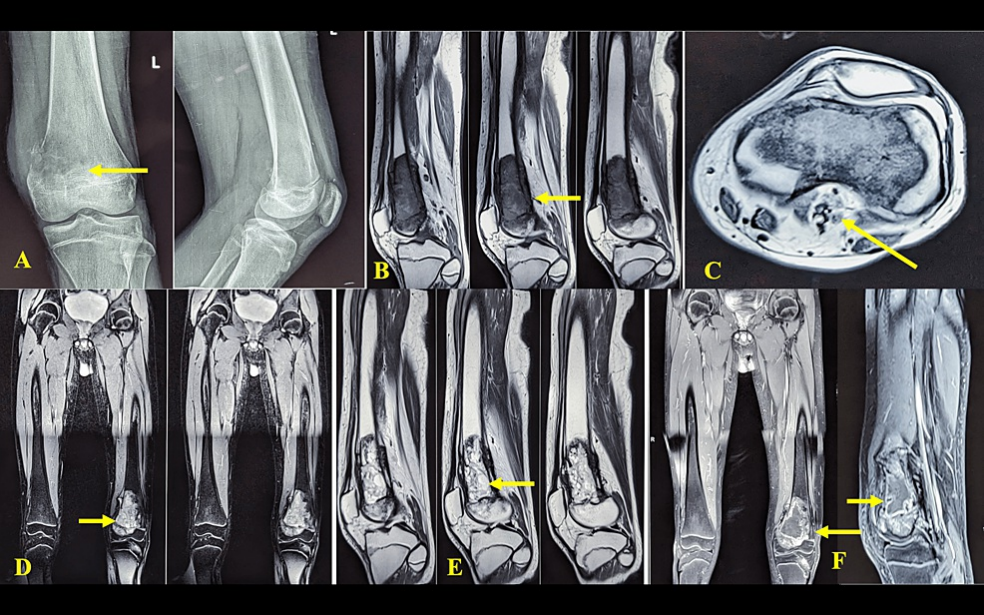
Pre-biopsy imaging evaluation: X-ray and MRI (plain and contrast). A: Plain X-ray of the left knee showing a lesion in the distal femur with a cortical break on the medial aspect. B: T1 sagittal section showing the lesion. C: T1 axial section showing the lesion and its relationship to the neurovascular bundle. D: T2 coronal section of the whole thigh including hip joints to rule out skip metastasis. E: T2 sagittal section showing the lesion. F: Contrast MRI images showing lesions in both coronal and sagittal sections.
